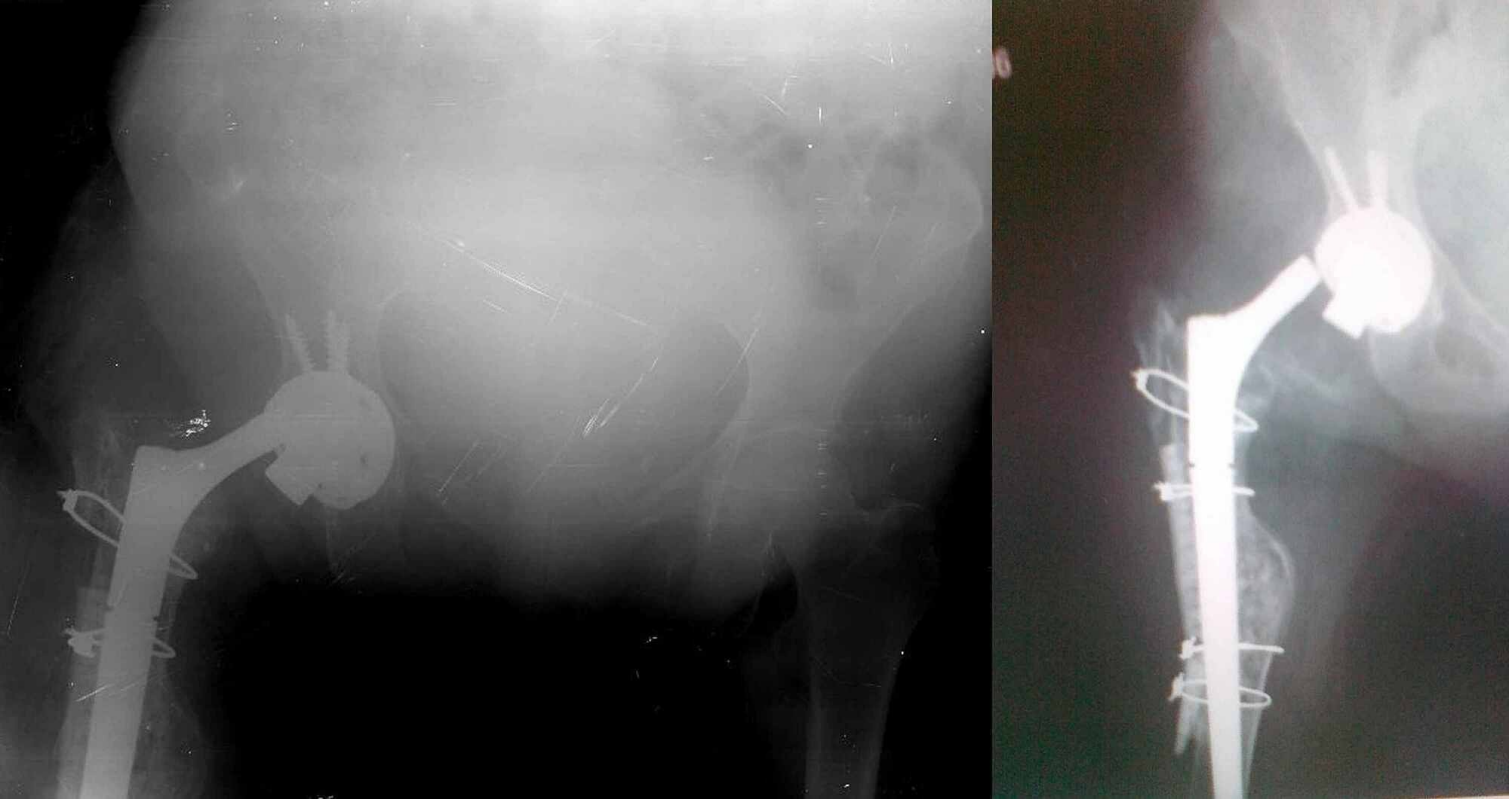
The direct radiographs of the patient three months after the surgery showing disassociation of the femoral head and neck components of the hip prosthesis.
